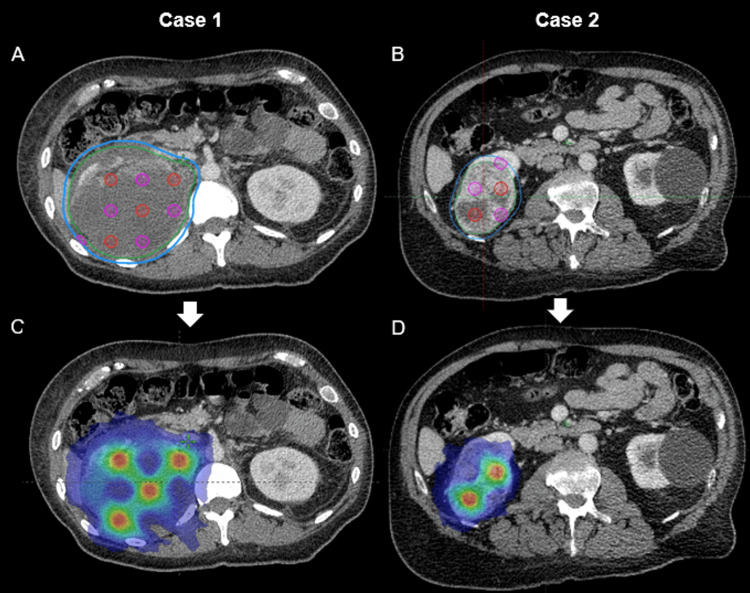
Case 1 and Case 2 - lattice radiotherapy plans. (A and B) Axial computed tomography slices of the targeted right renal mass, with green gross tumor volume prescribed 2000 cGy (GTV_2000) and blue planning tumor volume prescribed 2000 cGy (PTV_2000). Red spheres represent the 1.5-cm-diameter targets delineated as planning target volumes, boosted to 6670 cGy (PTV_6670). Pink spheres represent the avoidance structures delineated as PTV_Avoid. (C and D) Dose distribution after volumetric modulated arc therapy planning for the target (2000 cGy in blue, 6670 cGy in red).
